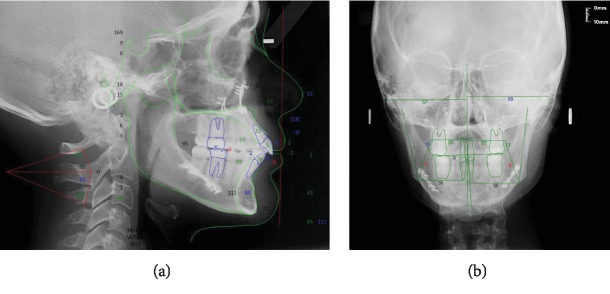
Cephalometric tracing (T4): (a) cephalometric evaluation of the lateral radiograph of the skull and (b) cephalometric evaluation of the posteroanterior radiograph of the skull; T4=follow-up (30 months after the end of the treatment).
